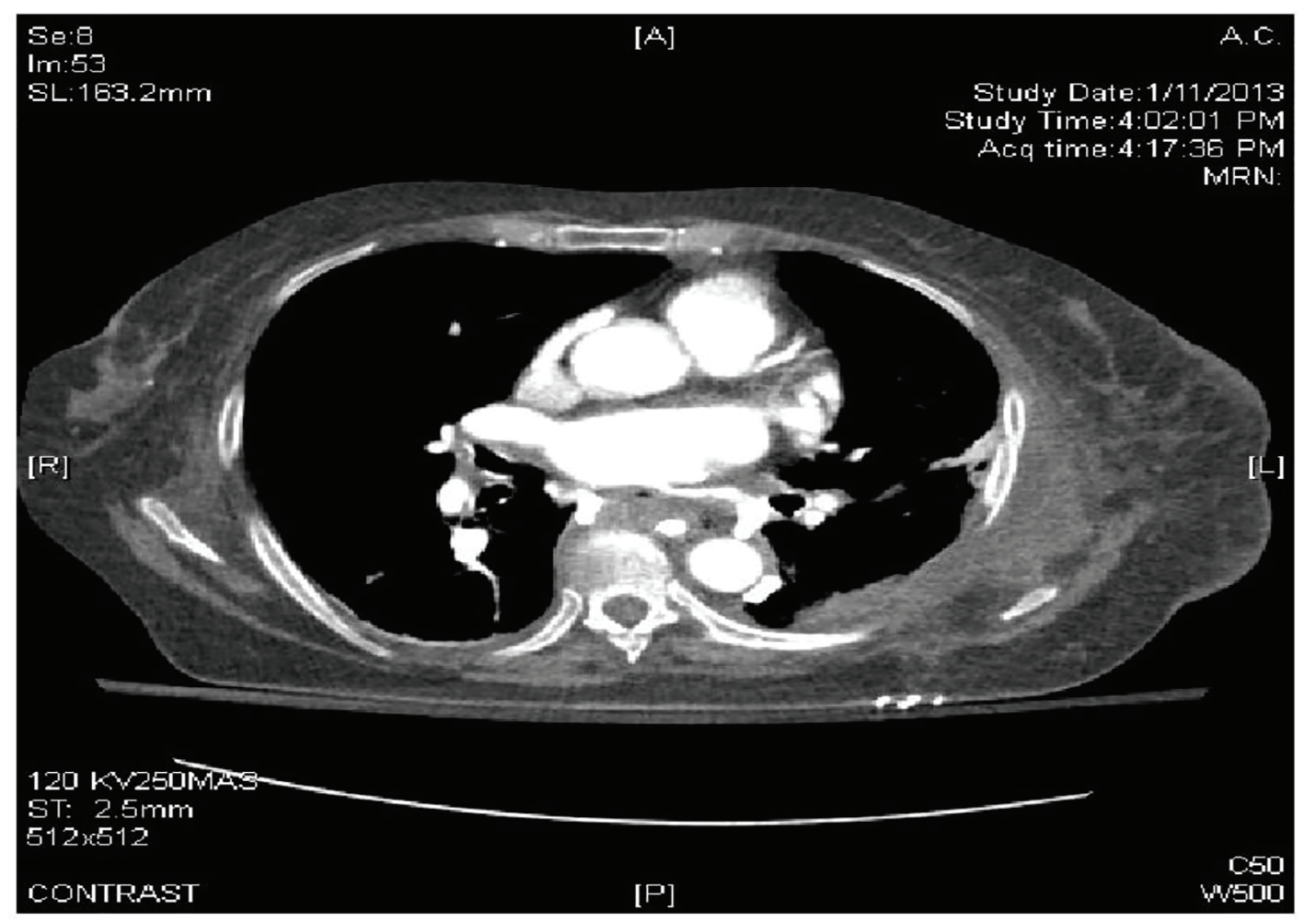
CTA of the chest and abdomen identified esophageal perforation with a fistulous tract to the left atrium.
